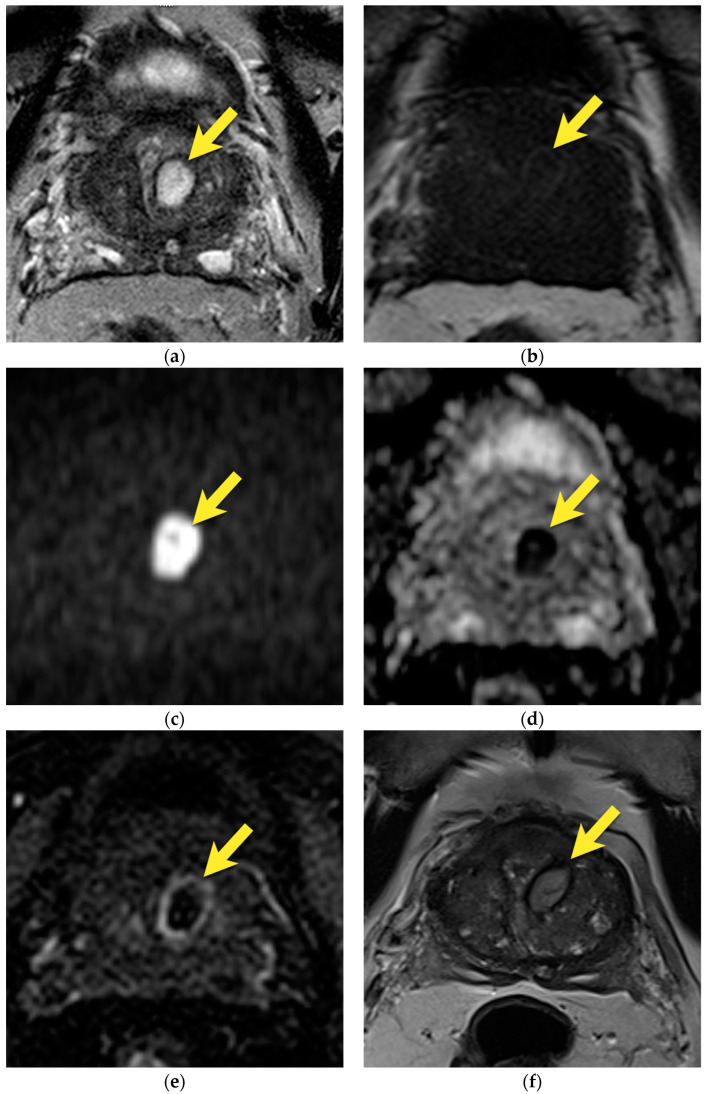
Abscess in the transition zone of the prostate: (a) axial T2-w image shows hyperintense, oval lesion in the left transition zone at the base of the prostate, with hypointense rim (arrow); (b) axial T1-w image shows isointense oval lesion, with slightly hyperintense rim (arrow); (c) with marked diffusion restriction high signal on DWI b 2000 (arrow); (d) corresponding to very low signal on ADC map (arrows); (e) rim enhancement on DCE (arrow); lesion was reported as PI-RADS 2; (f) after antibiotic treatment, the abscess persisted, but in T2-w images, its signal changed to intermediate intensity (arrow).
